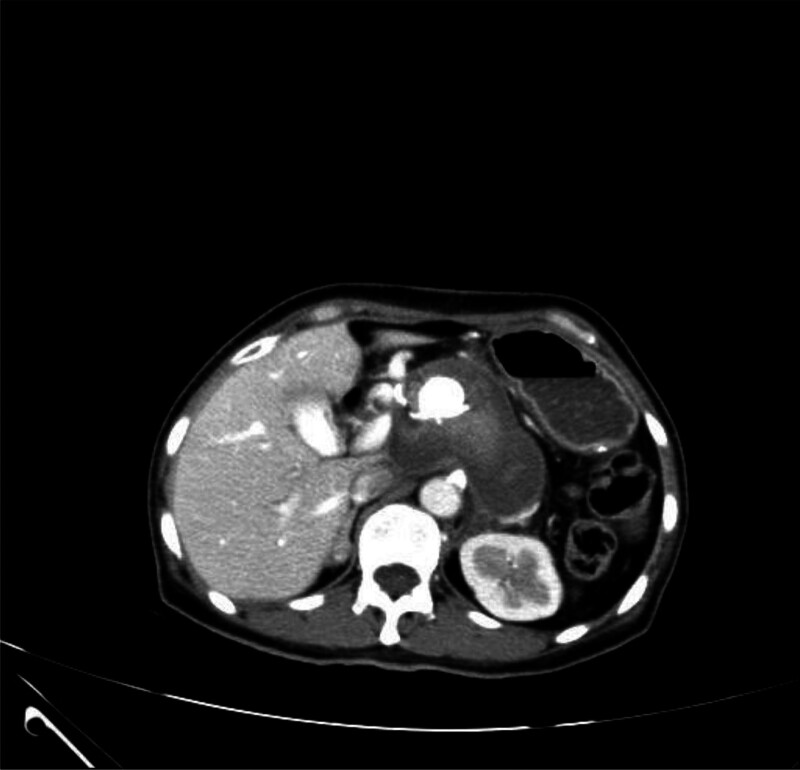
Postoperative CT showing no hemorrhage.
